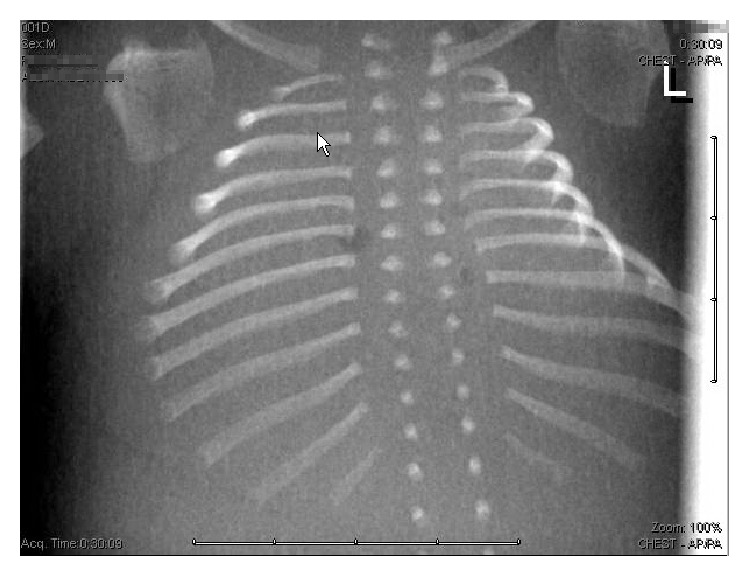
Postmortem radiograph showing complete absence of calcification of the vertebral bodies.
